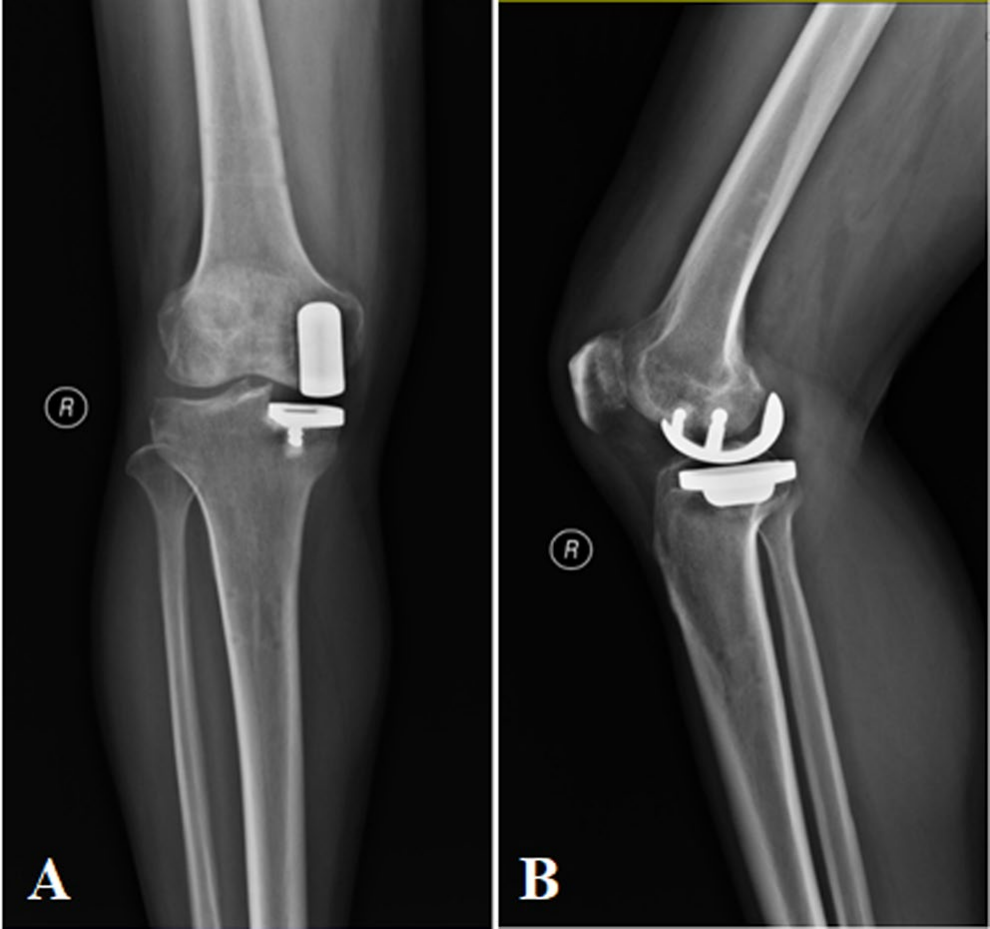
A, B The patient was followed up 8 months after surgery, and X-rays showed that the prosthesis was in good position without loosening
